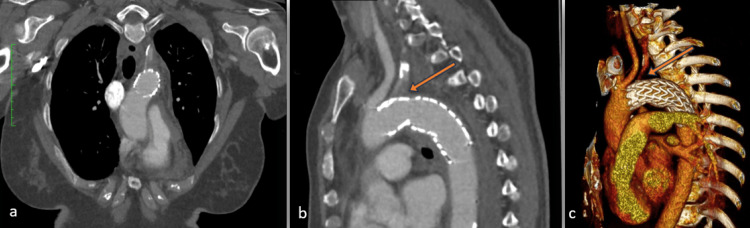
CT after TEVAR implantation: (a) transversal view, (b) sagittal view, and (c) 3D reconstruction The arrow indicates the occlusion of the left subclavian artery. TEVAR, thoracic endovascular aortic repair
